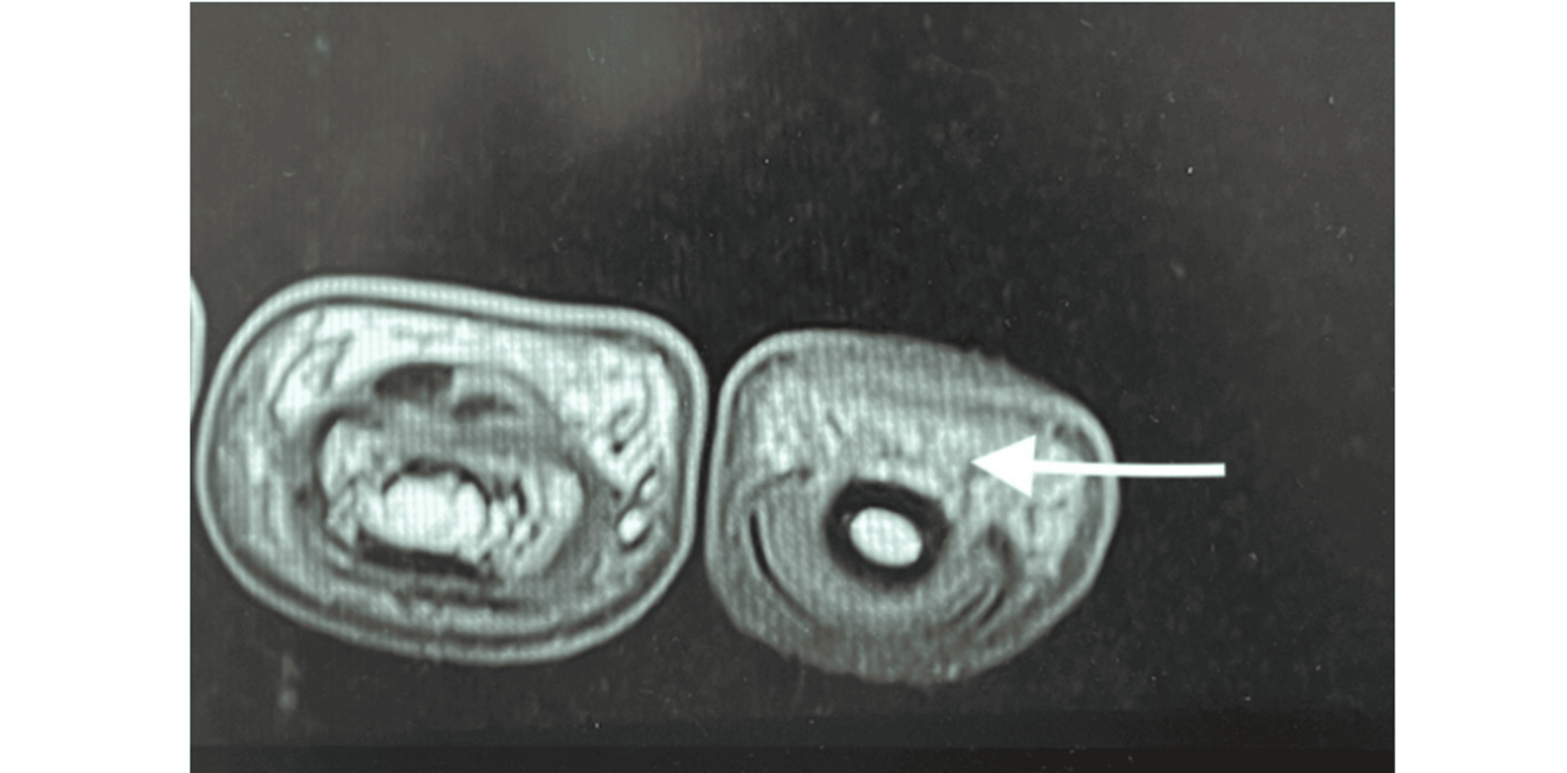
T1-weighted turbo spin echo sequence acquired in the transverse plane of magnetic resonance imaging at WL 413 and slice thickness 2.5 mm, showing absence of FDPt in index finger, at the level of distal phalanx. FDPt - flexor digitorum profundus tendon; WL - window level
